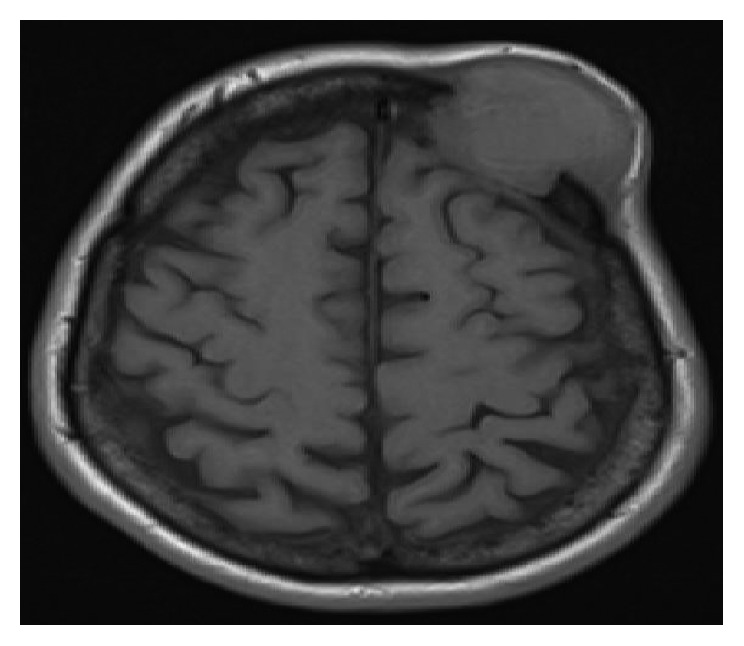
MRI brain showing calvarial mass lesion extending intracranially.
